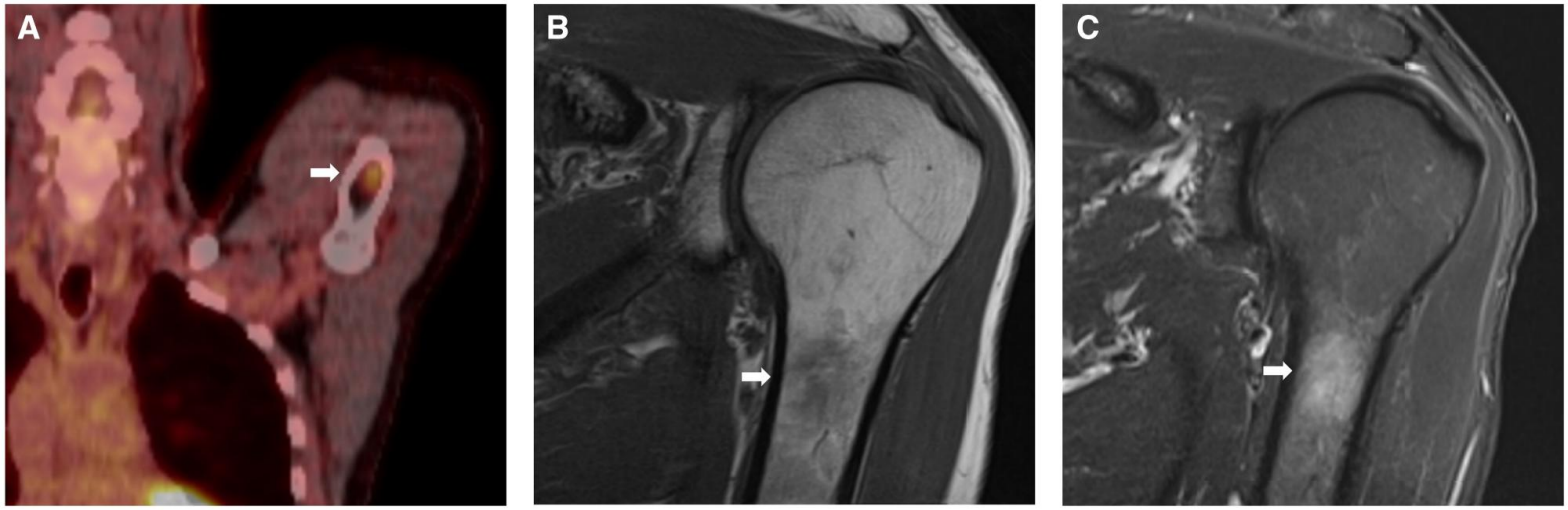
Left proximal humerus lesion (white arrow) in a 70-year-old male with a background of laryngeal malignancy. (A) FDG PET study demonstrated an avid lesion with an SUV of 1.9 (A). (B, C) Sagittal MRI sequences subsequently performed demonstrated reduced T1w signal (B) and increased STIR signal (C) within the lesion.
